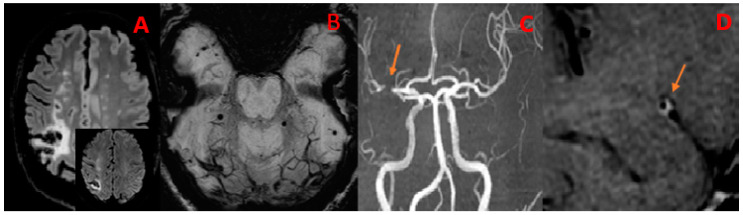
Patient (F/50) with a diagnosis of PCNSV. Ischemic stroke due to MCA M1 occlusion at the onset. (A) FLAIR T2-weighted image shows multiple WM alterations with ischemic lesions and a large chronic parietal infarct, with DWI hyperintensity. (B) SWI image shows multiple foci of bihemispheric microbleeding. (C) TOF MRA image shows loss of signal due to occlusion of the right M1 (arrow). (D) 3D SPACE T1-weighted VW image shows concentric, regular, and intense enhancement (arrow) of right M1 walls at the site of TOF alteration.
